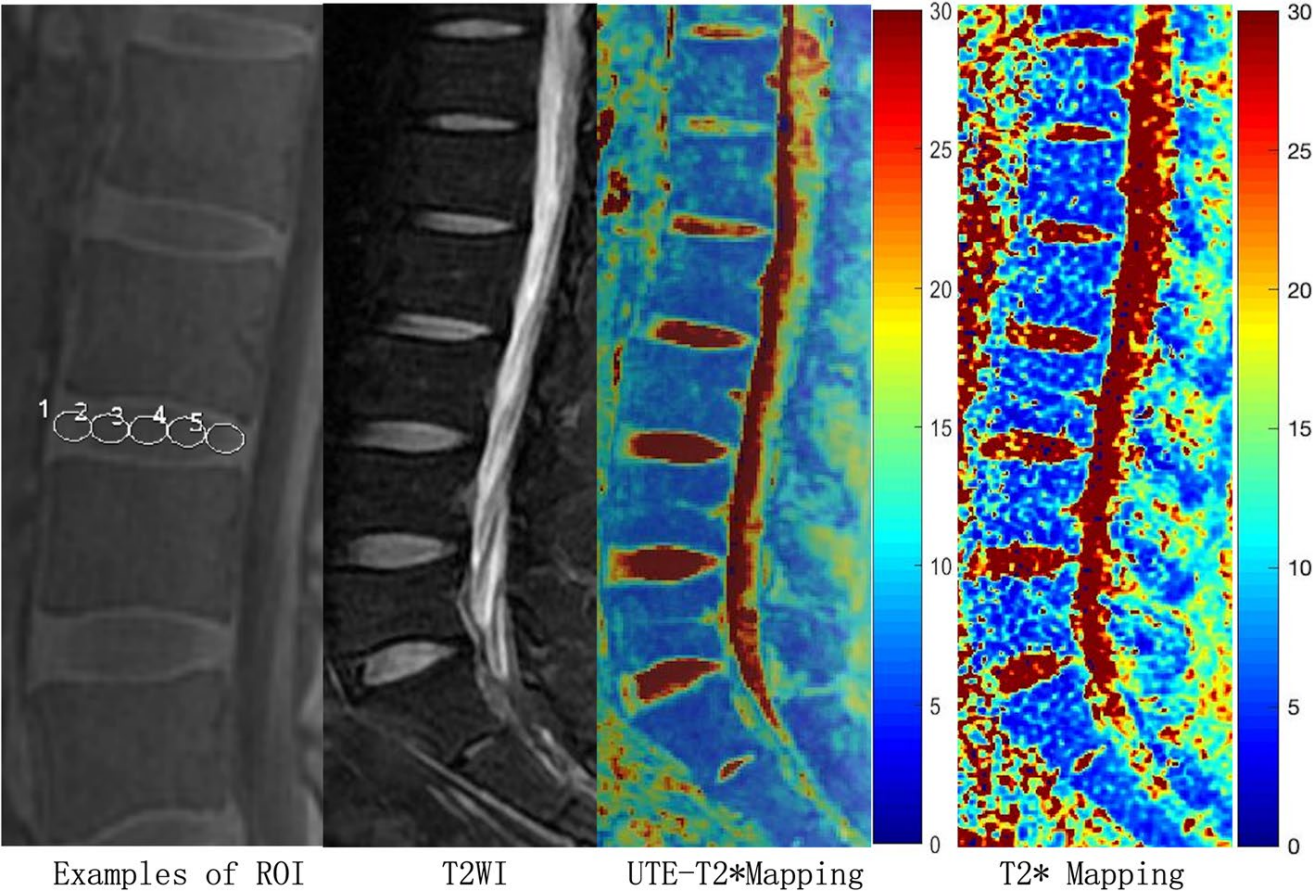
MR images of the lumbar spine of a 39-year-old woman. Every lumbar IVD was cut into 5 uniform parts in each UTE-T2* and T2* mapping. An ROI of 1 represented AAF, ROI 2–4 represented NP, and ROI 5 represented PAF. IVD, intervertebral disc; NP, nucleus pulposus; PAF, posterior annulus fibrosus; ROI, region of interest; UTE, ultrashort time-to-echo
